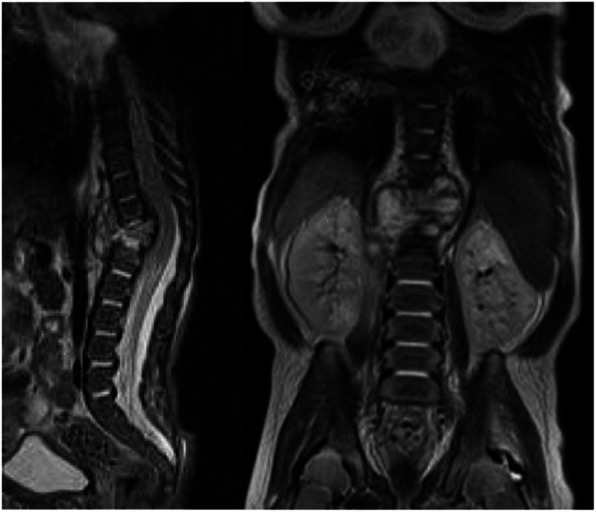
MRI at 5 weeks of age showing the destruction of the T12 vertebral body with involvement of disc between T12 and L1
